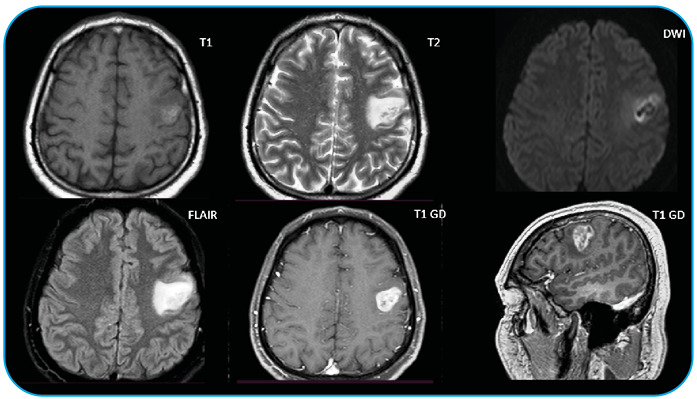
First MRI. Left to right, top to bottom, brain MRI sequences of axial slices in unenhanced T1, T2, diffusion, FLAIR, T1 with contrast, and sagittal T1 with contrast, respectively. Lesions pointed by blue arrows.
